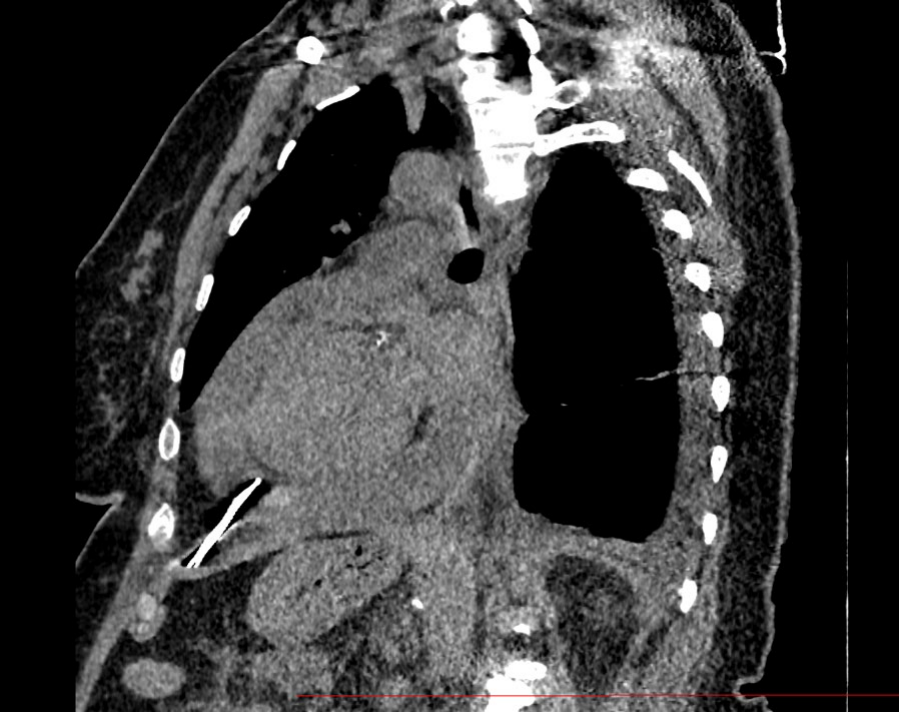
Sagittal view of a preoperative thoracic CT scan centered on the Kirschner wire without contrast
